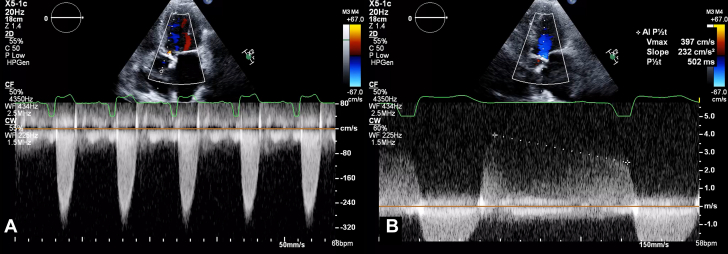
Apical 5-Chamber View Continuous- Wave Doppler Assessment Apical 5 chamber: align CW Doppler through the aortic valve to obtain aortic valve peak velocity (A) and gradients and Doppler assessment of aortic regurgitation (B).
